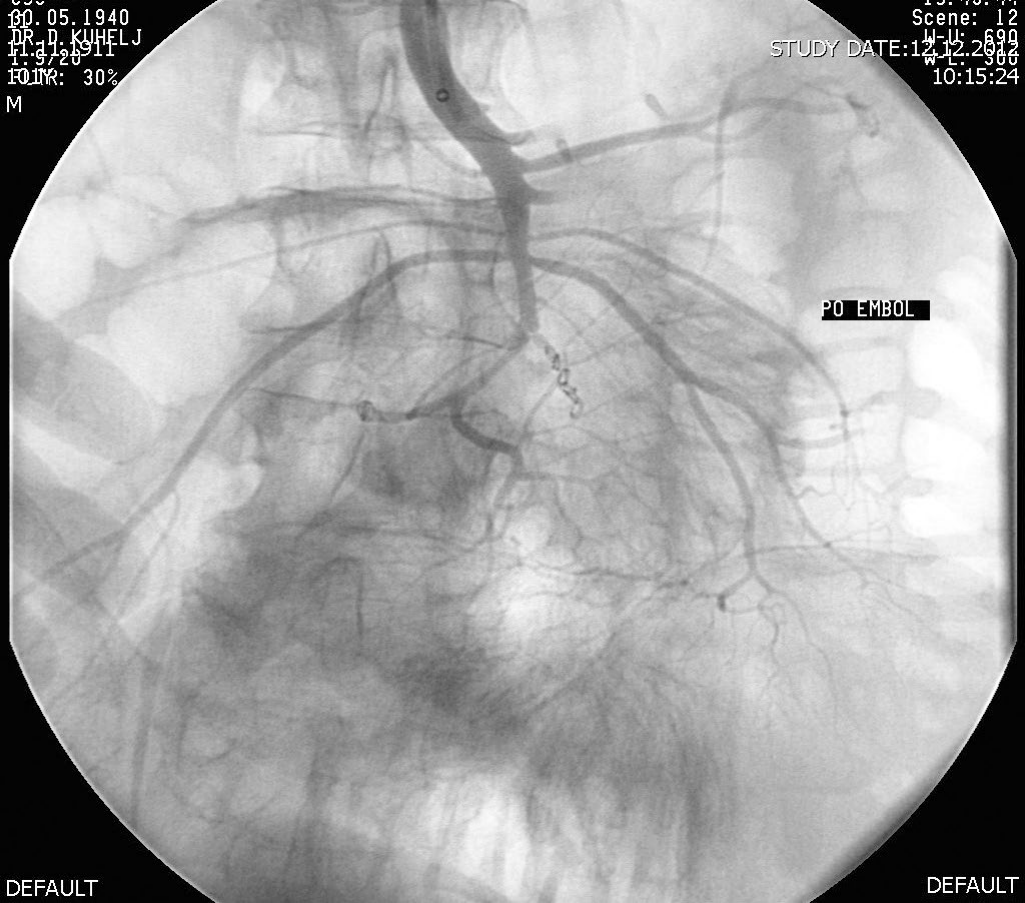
Doppler US control 5 days after the treatment showing patent SMA.
